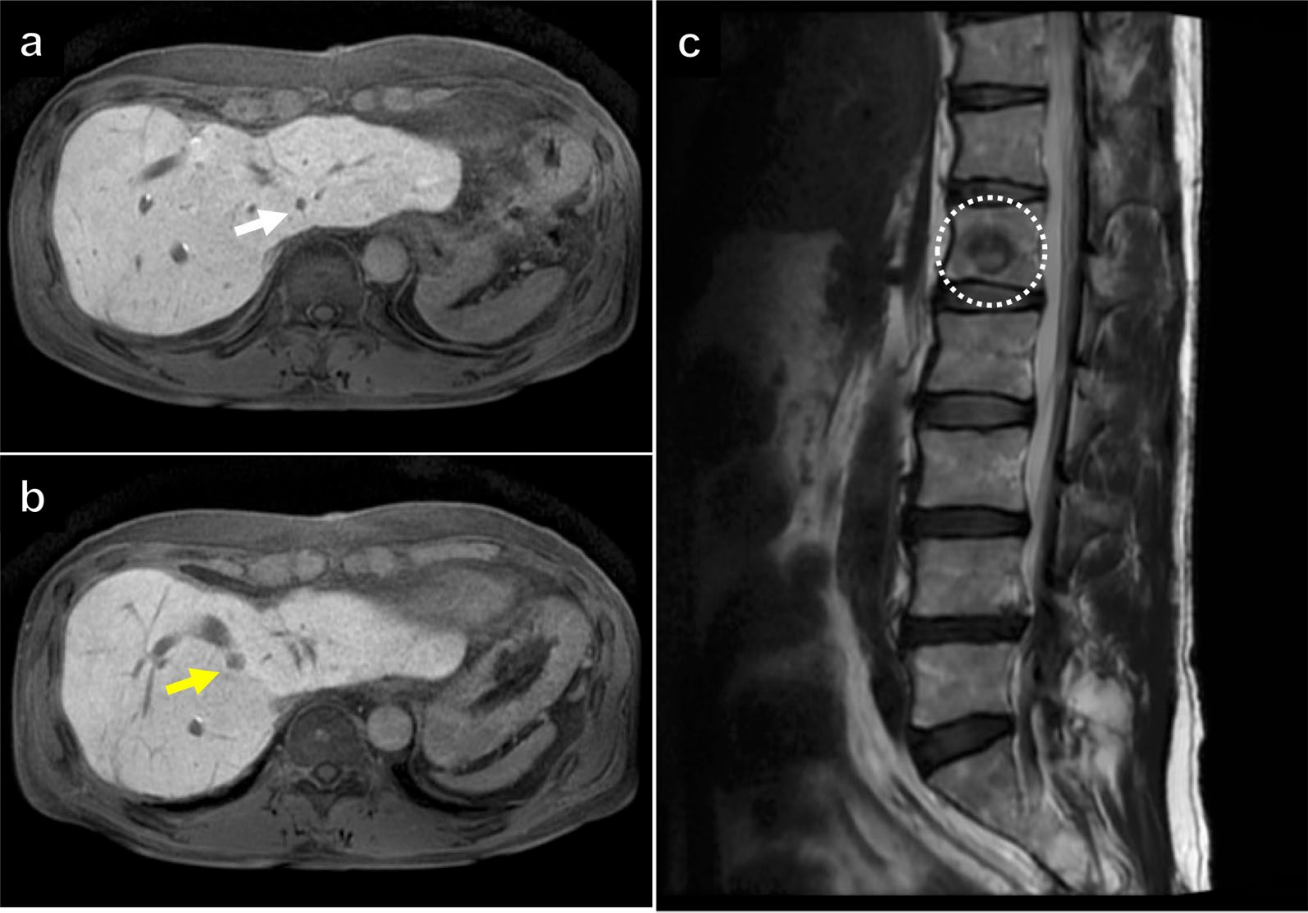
Gadolinium-ethoxybenzyl-diethylene-triamine-pentaacetic acid enhanced magnetic resonance imaging revealed only two liver metastases in segment 2 (a white arrow) and 8 (b yellow arrow). The bone metastasis had no remarkable change in size (c white dotted circle)
